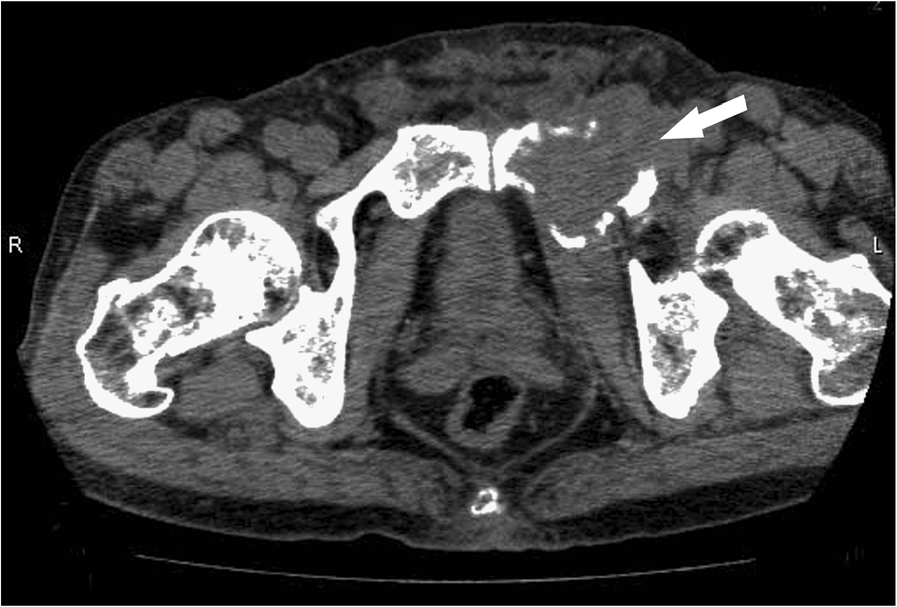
Axial computed tomography of the pelvis demonstrating a solitary, lytic, expansile lesion in the left superior pubic ramus.
